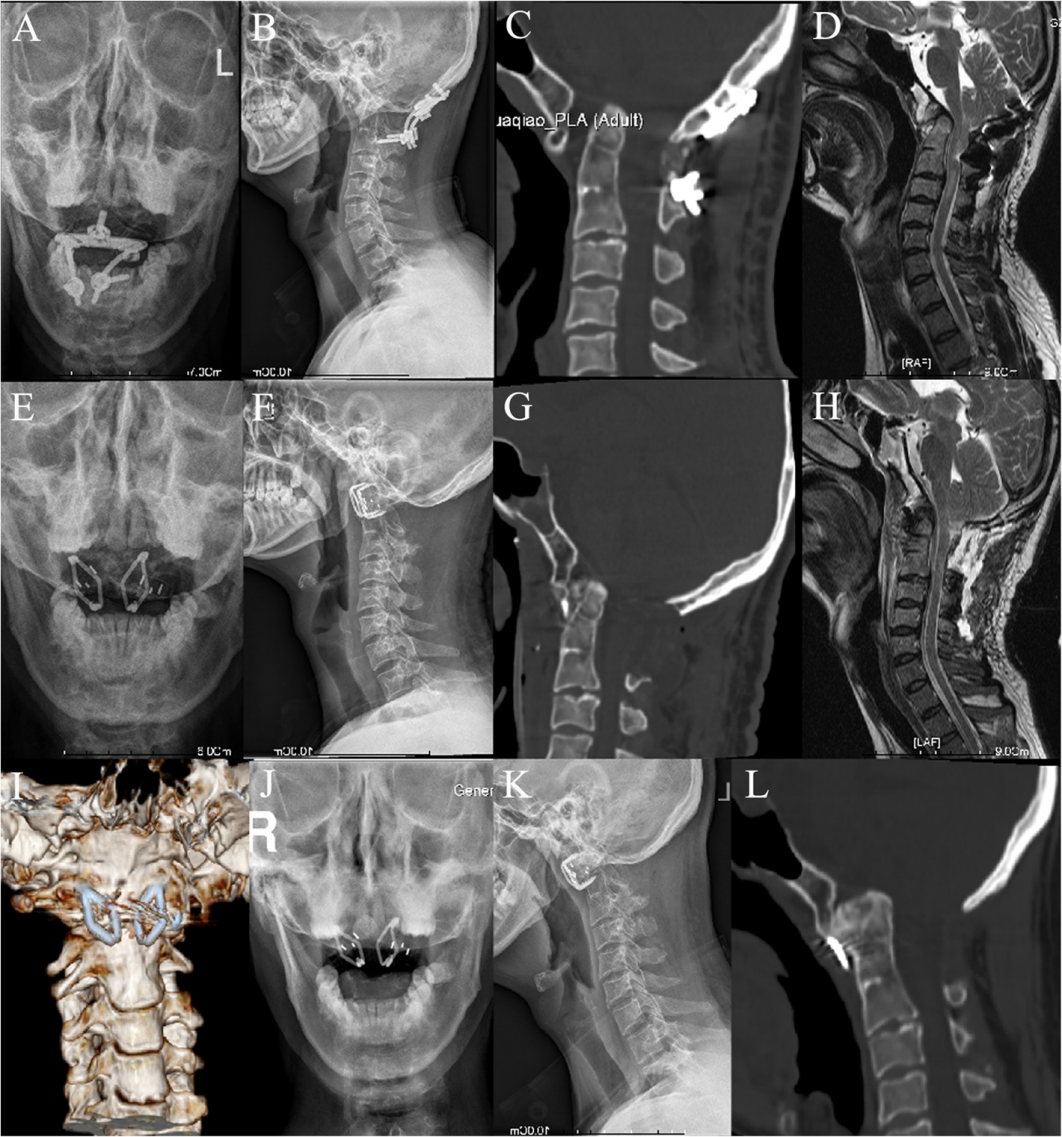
A 41-year-old man was diagnosed with BI with IAAD after a failed posterior OCF, and revised by transoral intraarticular cage distraction and C-JAWS fixation. a-c Images of cervical X-rays and CT scan before revision surgery showed evidence of BI with IAAD. d Preoperative Sagittal MRI showed compression of the cervicomedullary junction. e-g Postoperative cervical X-rays and CT scan performed at 1 week after revision surgery showed satisfactory reduction and fixation. h Postoperative sagittal MRI showed a desirable decompression of the cervicomedullary junction. i Postoperative three-dimensional reconstruction showed location of C-JAWS. j-l Cervical X-rays and CT scan at 6-month follow-up showed a solid bone fusion without loss of reduction
